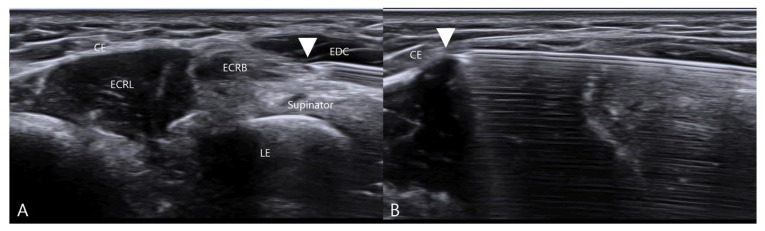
Real-time ultrasound-guided distal-to-proximal in-plane needle advancement and injectate delivery. (A) A 23-gauge, 6 cm needle was introduced from a distal skin entry point under real-time in-plane ultrasound guidance. The needle was oriented proximally and maintained nearly parallel to the skin surface and the long axis of the humerus. (B) The needle tip was advanced to the superficial fascial plane overlying the common extensor aponeurosis (CE) under continuous sonographic visualization before injectate delivery. The intended target plane was located superficial to the CE and adjacent to the PABCN-related fascial plane. Arrowhead, needle tip; CE, common extensor aponeurosis; LE, lateral epicondyle; ECRL, extensor carpi radialis longus; ECRB, extensor carpi radialis brevis; EDC, extensor digitorum communis.
